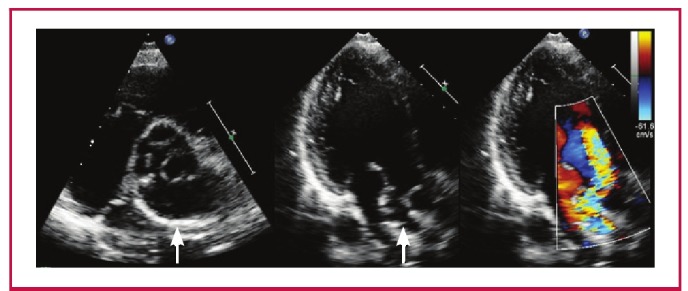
Parasternal short-axis view of the sub-aortic (SA) aneurysm (white arrow, left). Apical three-chamber views depicting the SA aneurysm (white arrow, middle), and an eccentric aortic regurgitation jet on colour flow, with flow into the SA aneurysm (right).
